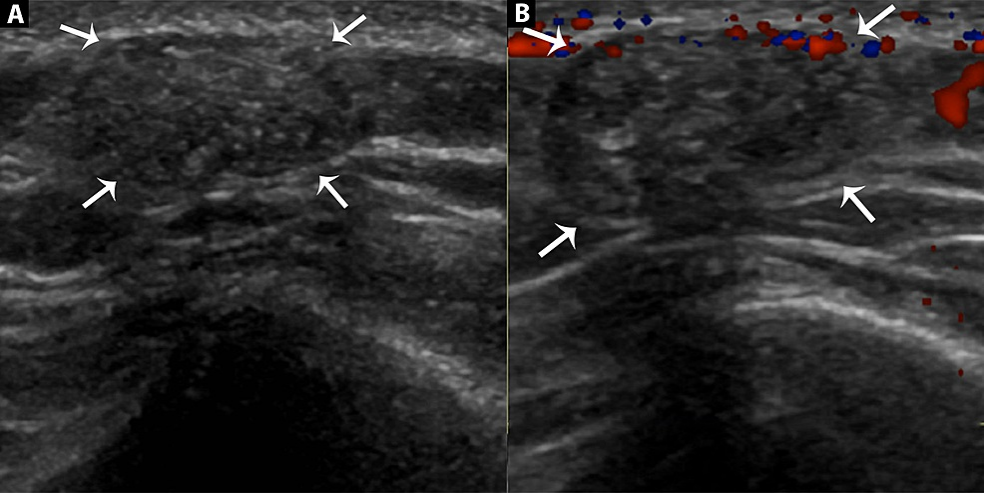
Ultrasound of the right shoulder (A) Greyscale ultrasound of shoulder showing, an oval-shaped, well-defined isoechoic lesion, having hypoechoic margins (white arrows) and internal calcifications mainly in the peripheral regions. It is associated with focal thinning of subcutaneous fat plains. (B) Doppler ultrasound showing the absence of vascularity within the lesion, consistent with the benign nature of the swelling.
